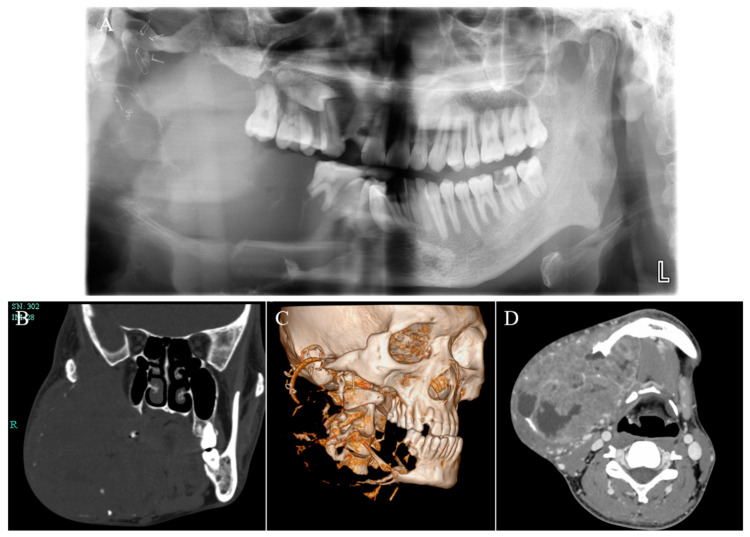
Panoramic radiography (A), CT (B), three-dimensional reconstruction (C), and MRI (D) showed an expansive growth pattern of the tumor with bony destruction and huge soft tissue mass.
